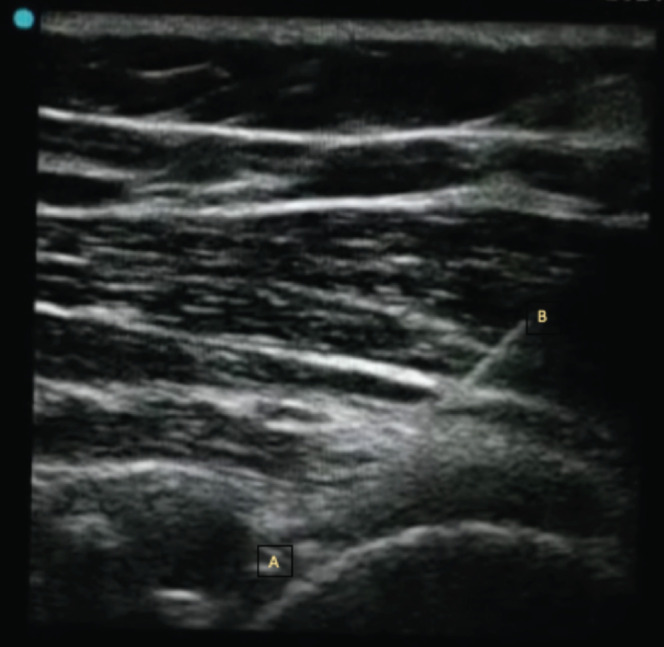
Hydrodilatation with guidance of ultrasound in the glenohumeral capsule by the posterior approach (transverse view), A: Glenohumeral joint, B: Injecting Needle.
